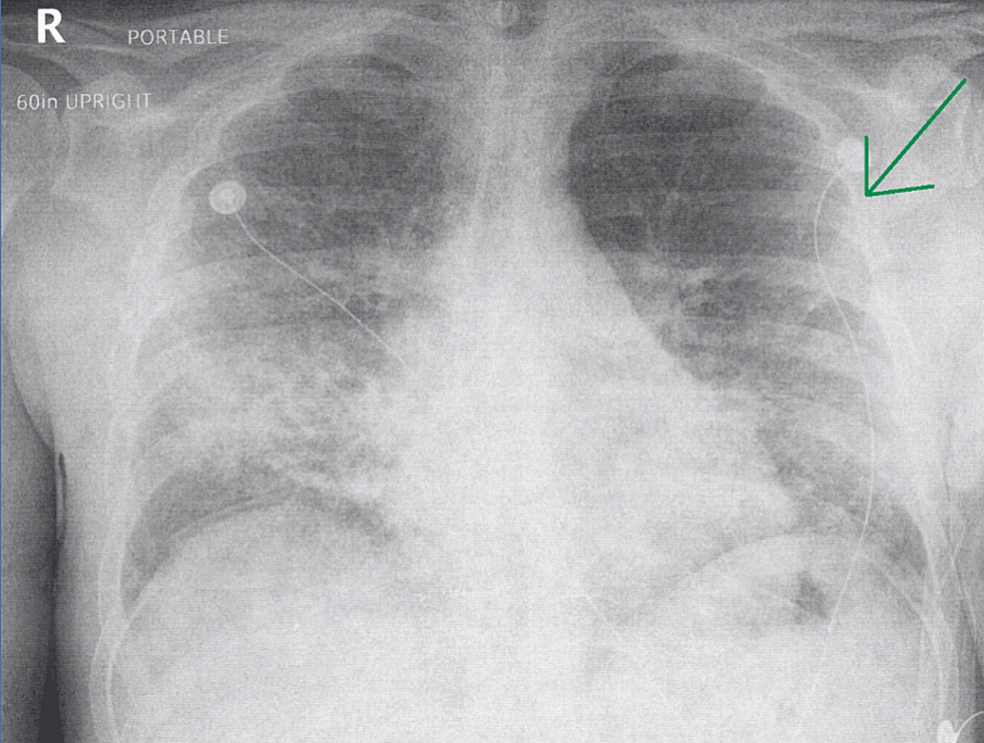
Anterior-posterior chest X-ray on repeat admission
